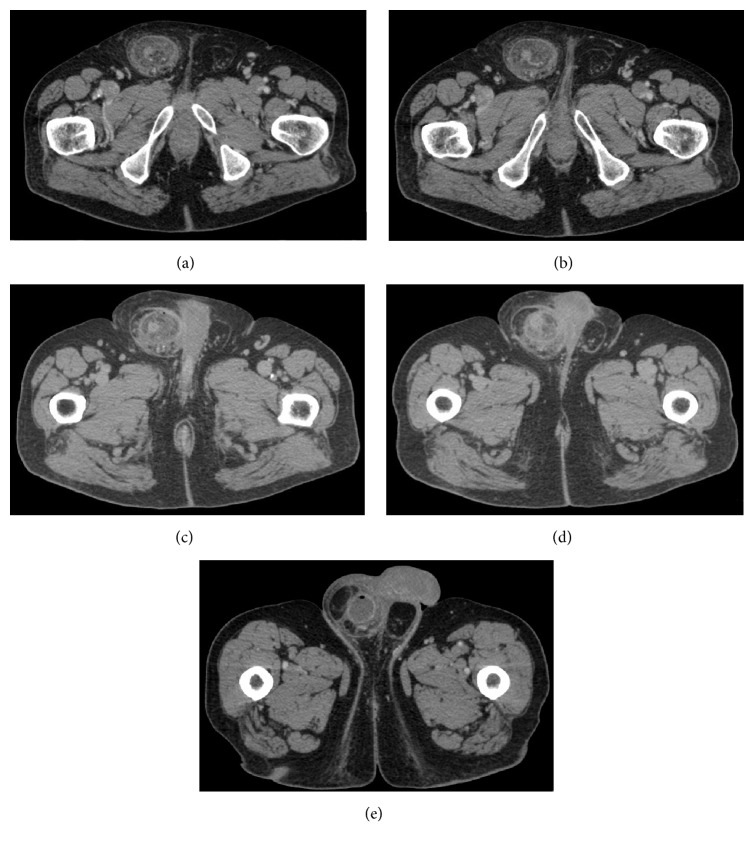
Inguinal hernia with incarceration of a part of cecum and vermiform appendix at CT scan with endovenous contrast.
